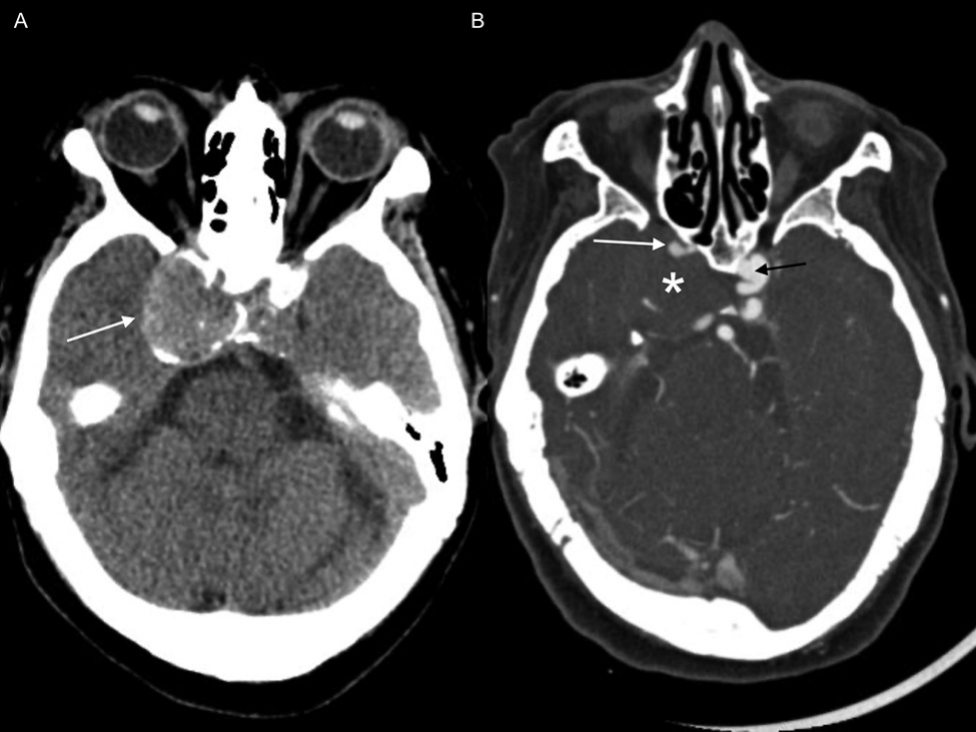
Unenhanced axial computed tomography (CT) scan (
